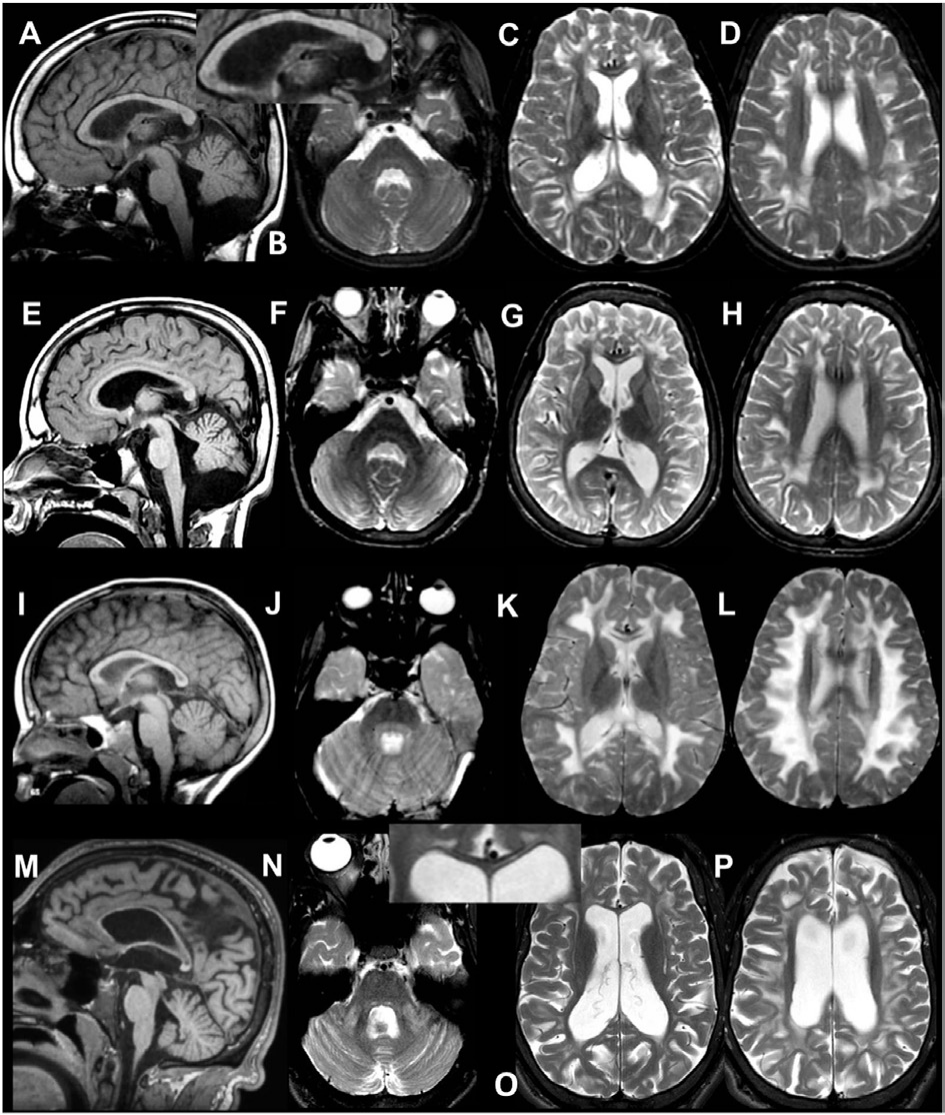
MRI of patient 1.1 at 9 years (A–D) and 12 years (E–H). The first MRI shows signal abnormalities in the middle blade of the corpus callosum [(A,C) and inset in (A)]; inhomogeneous mainly multifocal signal abnormalities in the subcortical and deep cerebral white matter, sparing a periventricular rim (C,D); and slight cerebral (C,D) but no cerebellar atrophy (A,B). At follow-up, slightly increased cerebral and cerebellar atrophy is seen. MRI of patient 2 at 4 years (I–L) and 20 years (M–P). The first MRI shows signal abnormalities in the middle blade of the corpus callosum (I,K), inhomogeneous, mainly confluent signal abnormalities in the subcortical and deep cerebral white matter, sparing a periventricular rim (K,L) and no cerebral or cerebellar atrophy (I–L). At follow-up, severe cerebral and milder cerebellar atrophy is seen (M–P). The corpus callosum is highly atrophic, but the selective involvement of the middle blade is still seen [inset in (O)]. Within the posterior fossa, signal abnormalities are present in the middle cerebellar peduncles and pontine central tegmental tracts (N).
